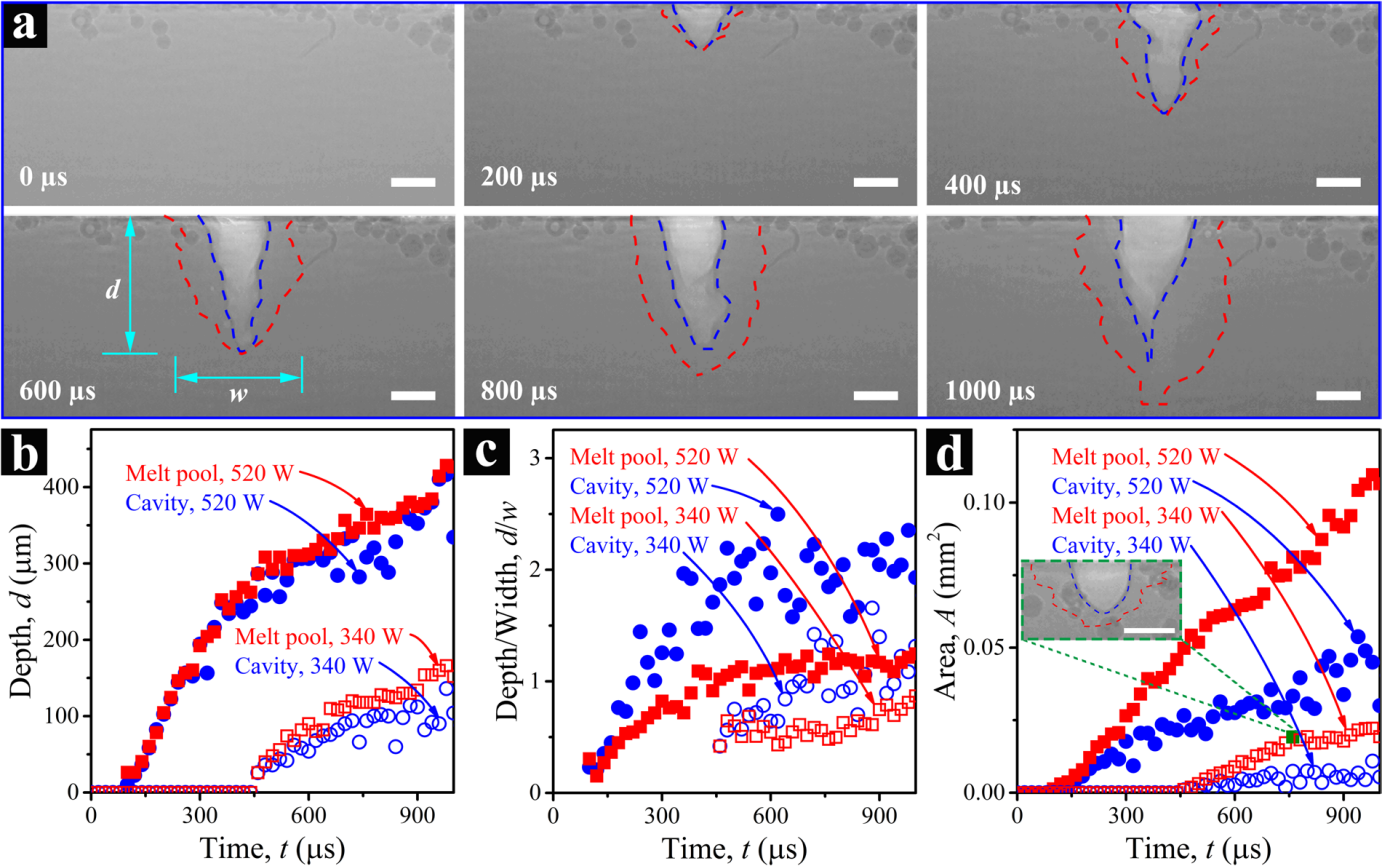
Dynamic evolution of the melt pool in laser powder bed fusion processes of Ti-6Al-4V. (a) X-ray images of melt pools in the metal base at *t* = 0, 200, 400, 600, 800, and 1000 µs, respectively. The blue dashed line marks the boundary of the cavity or depression zone, and the red dashed line indicates the liquid-solid interface. The power and size of the laser beam are 520 W and ~220 µm (1/e^2^), respectively. The laser is turned ON at *t* = 0, and continues to heat the sample till *t* = 1000 µs. The powder particle size is in the range of 5–45 µm, and the powder layer thickness is ~100 µm. (b–d) Development of the melt pool size and geometry as a function of laser heating time under two different laser power conditions: (b) depth, (c) aspect ratio (depth/width), (d) nominal area. The inset to (d) shows a steady-state melt pool configuration at the time indicated by the solid green square. All the scale bars in (a) and in the inset to (d) are 100 µm.
